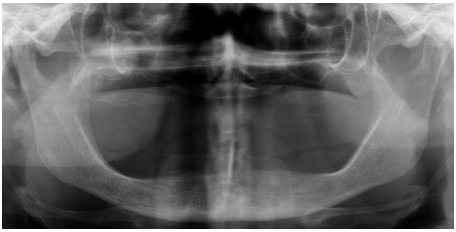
Panoramic radiographic after one year disclosing no recurrence of the peripheral osteoma on left the zygomatic arch.
